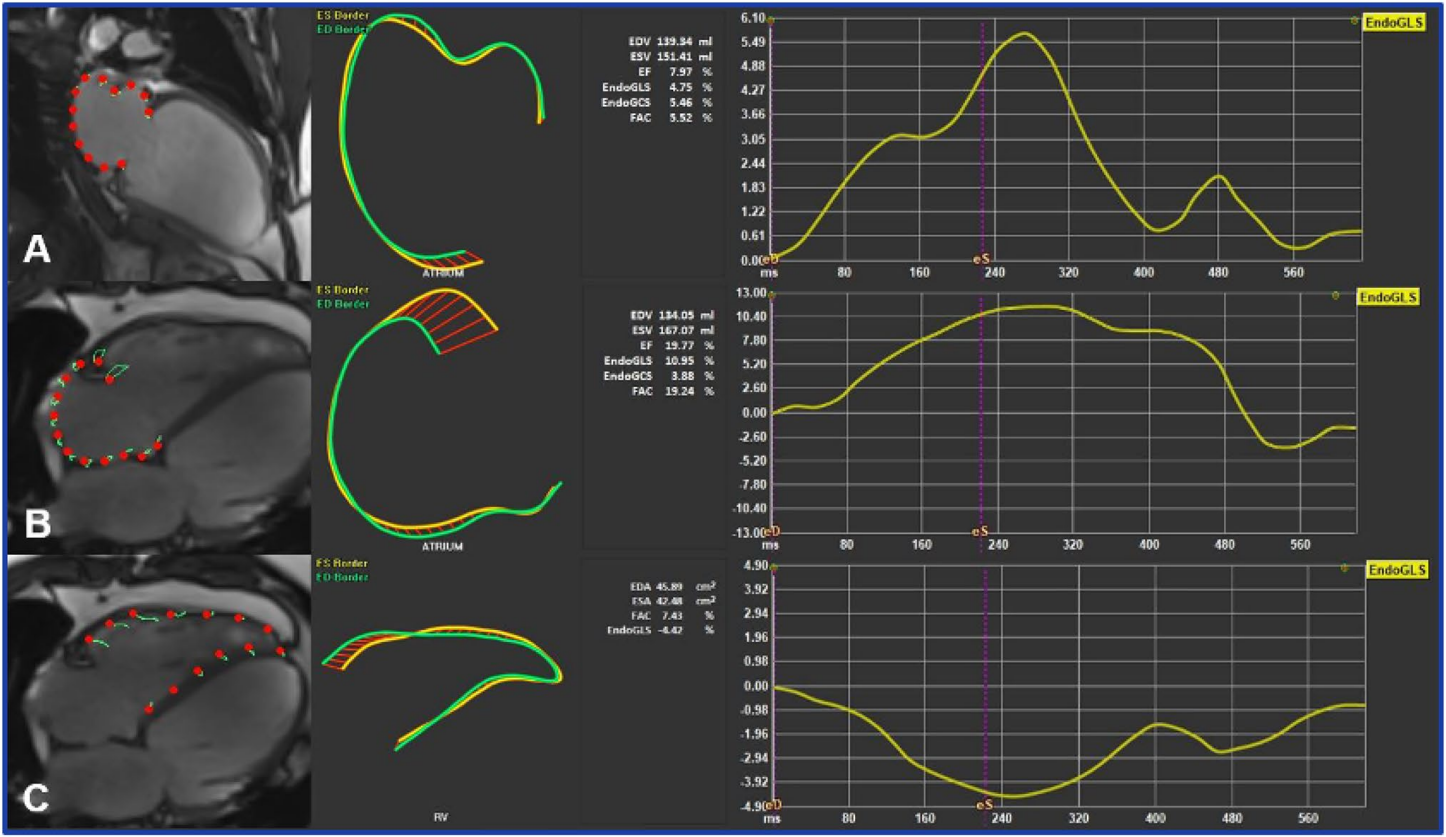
Cardiac magnetic resonance imaging-derived whole-heart myocardial mechanical parameters assessment using feature tracking software. Global longitudinal strain of the left atrium A right atrium B and right ventricle C was assessed by tracing endocardial borders on long-axis SSPF cine images in end-systole and end-diastole
